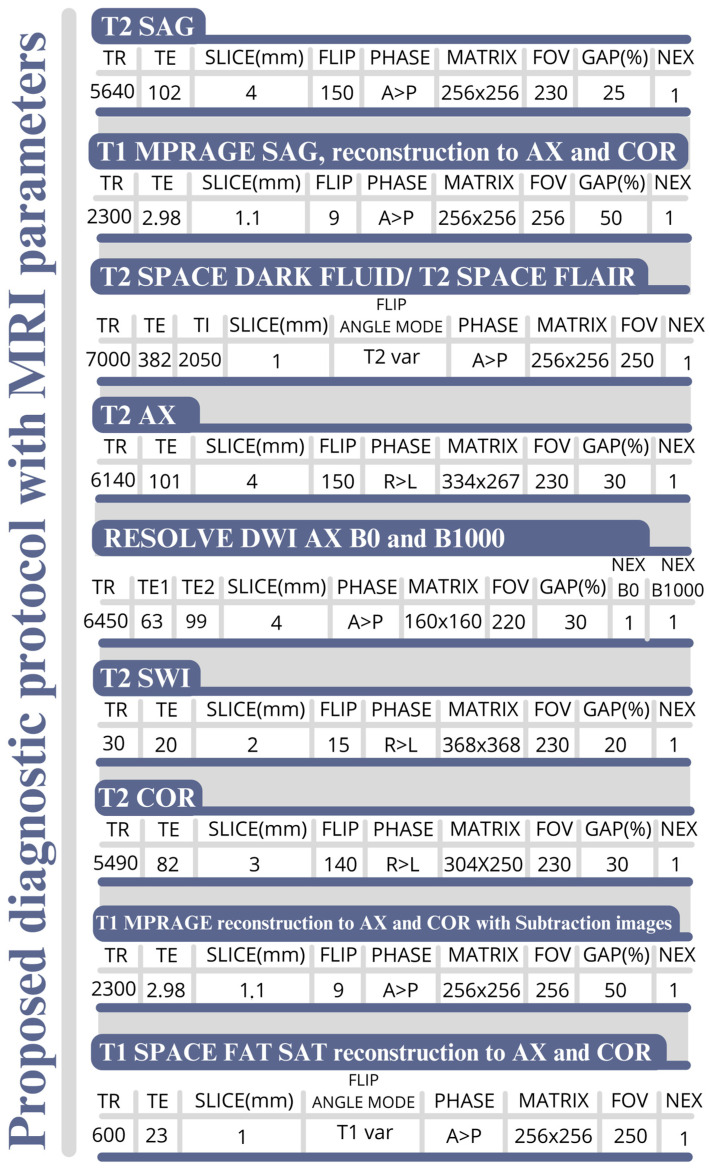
Proposed MRI protocol for demyelinating diseases and encephalitis—TOTAL SCAN TIME (3T MRI) = 26 min 20 s. (AX—axial, COR—coronal, DWI—diffusion-weighted imaging, FAT SAT—Fat Saturation, FLAIR—Fluid-Attenuated Inversion Recovery, FOV—field of view, MPRAGE—Magnetization Prepared Rapid Gradient Echo, NEX—number of excitation, RESOLVE—Readout SEgmentation Of Long Variable Echo-trains, SAG—sagittal, SPACE—sampling perfection with application-optimised contrasts using different flip-angle evolutions, SWI—Susceptibility-weighted imaging, TI—inversion time, TR—repetition time).
